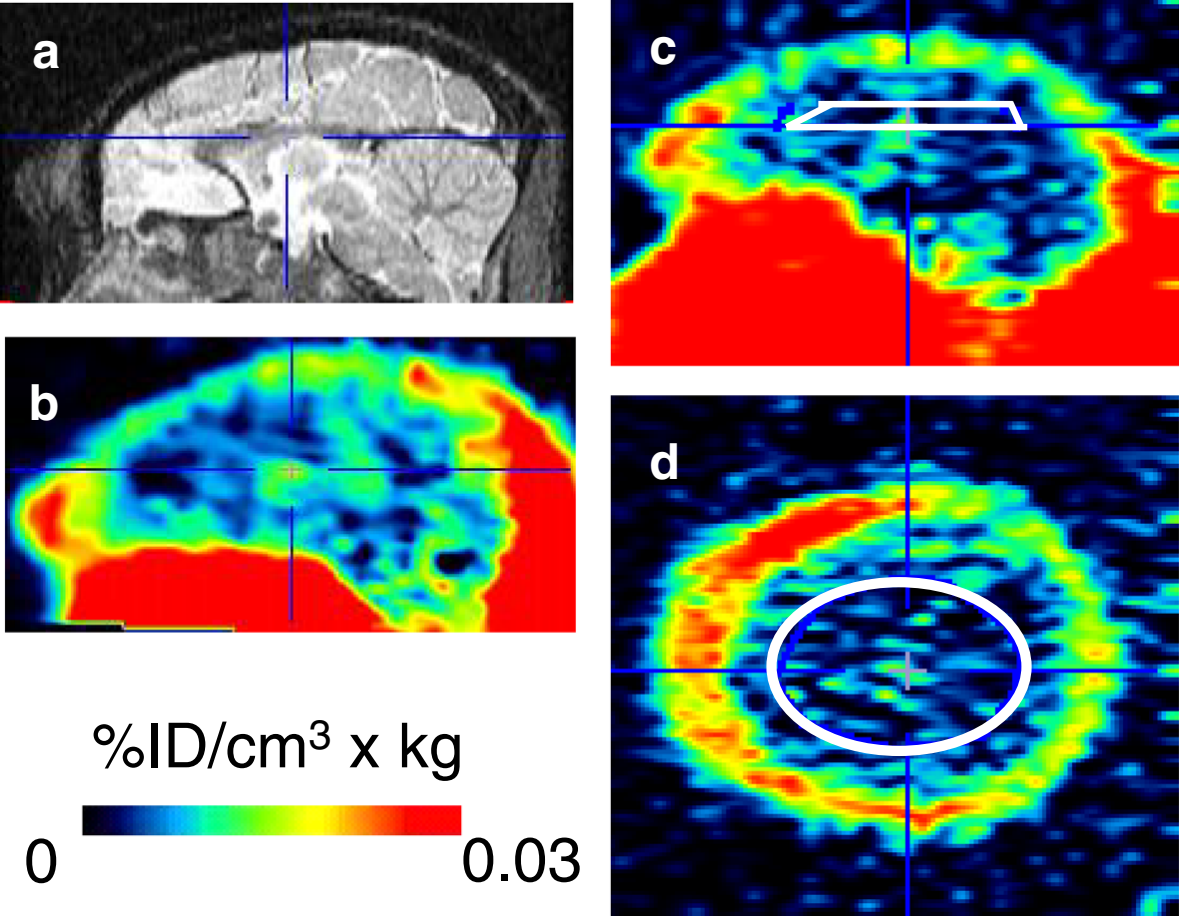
MR and PET images. Sagittal planes of MRI (a) and the coregistered averaged PET image from 10 to 60 min after [^11^C]oseltamivir injection (b) and region of interest (c, d).
